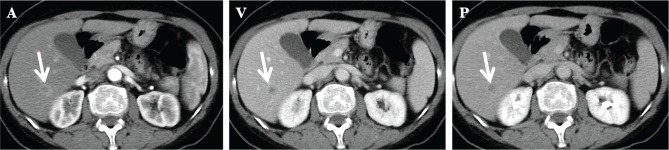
CT abdomen showing an ill-defined low-density area in sg VI with hyper-enhancement in the early phase and iso-enhancement in the late phase A – arterial phase; V – venous phase; P – Portal phase.
